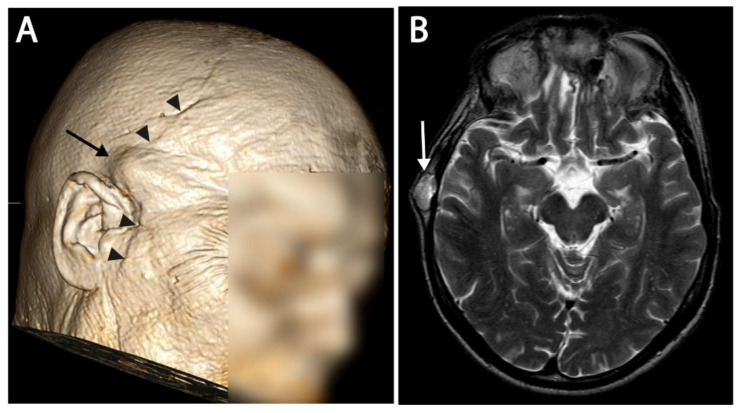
(A) 3D reconstructed image of an MRI. A subcutaneous recurrence is visible as a bump under the skin, and is indicated with an arrow. The surgical scar from the coronal midfacial approach is also visible and marked with arrowheads. (B) T2 image of the same patient at the level of recurrence. Subcutaneous recurrence is marked with an arrow.
